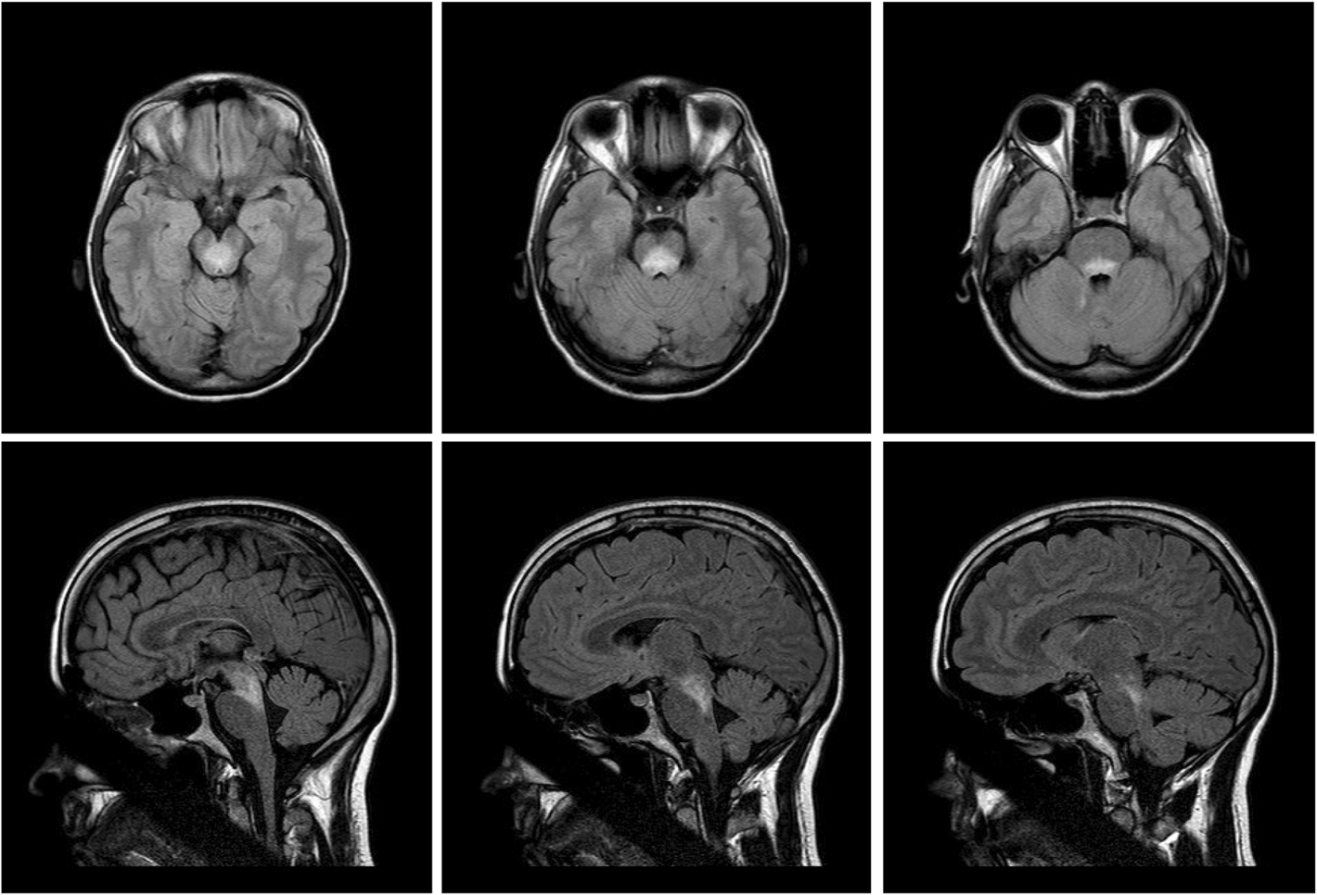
Axial (upper row) and sagittal (lower row) MRI of the brain. Gadolinium enhanced MRI with FLAIR revealed homogeneous hyperintensity involving the midbrain
